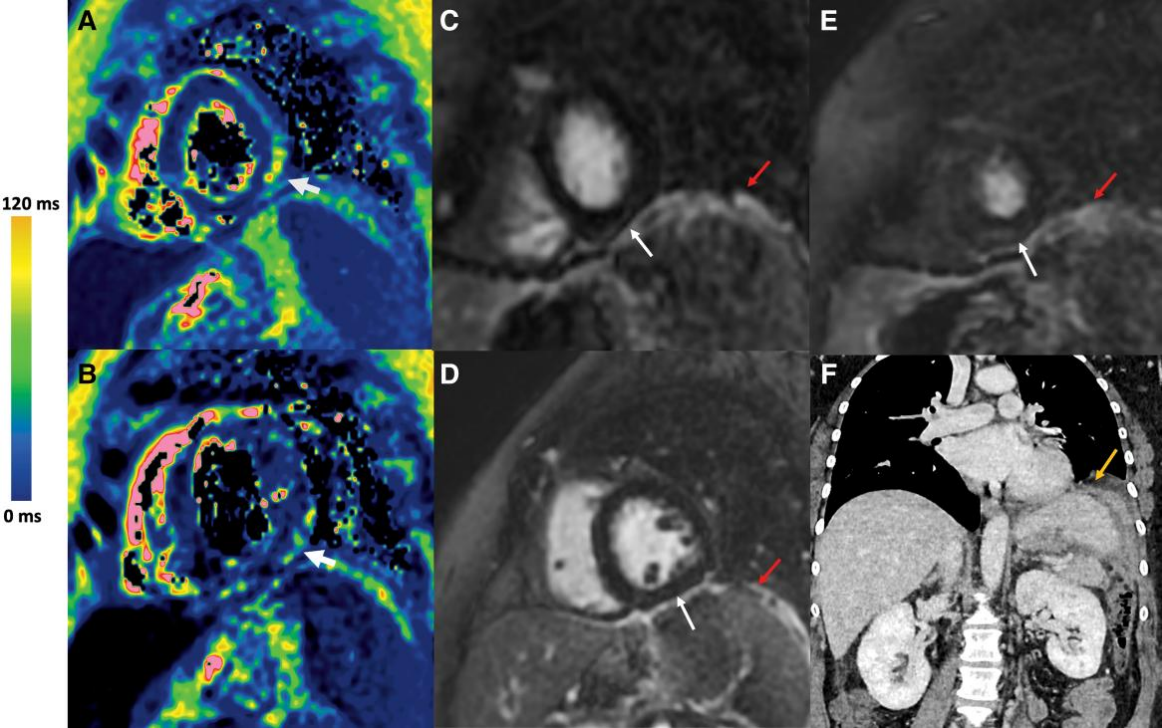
Myocarditis with peritoneal lymphoma carcinosis. (*A* and *B*) T2 mapping sequence (CMR). Presence of a T2 elevation in the subepicardial region of the inferolateral wall (white arrow). (*C*–*E*) LGE sequence (CMR). Presence of a subepicardial enhancement in the inferolateral region (white arrow). Visualization of a hyperintense thickening due to peritoneal lymphoma carcinomatosis (red arrow). (*F*) CT scan showing peritoneal lymphoma carcinomatosis (orange arrow).
